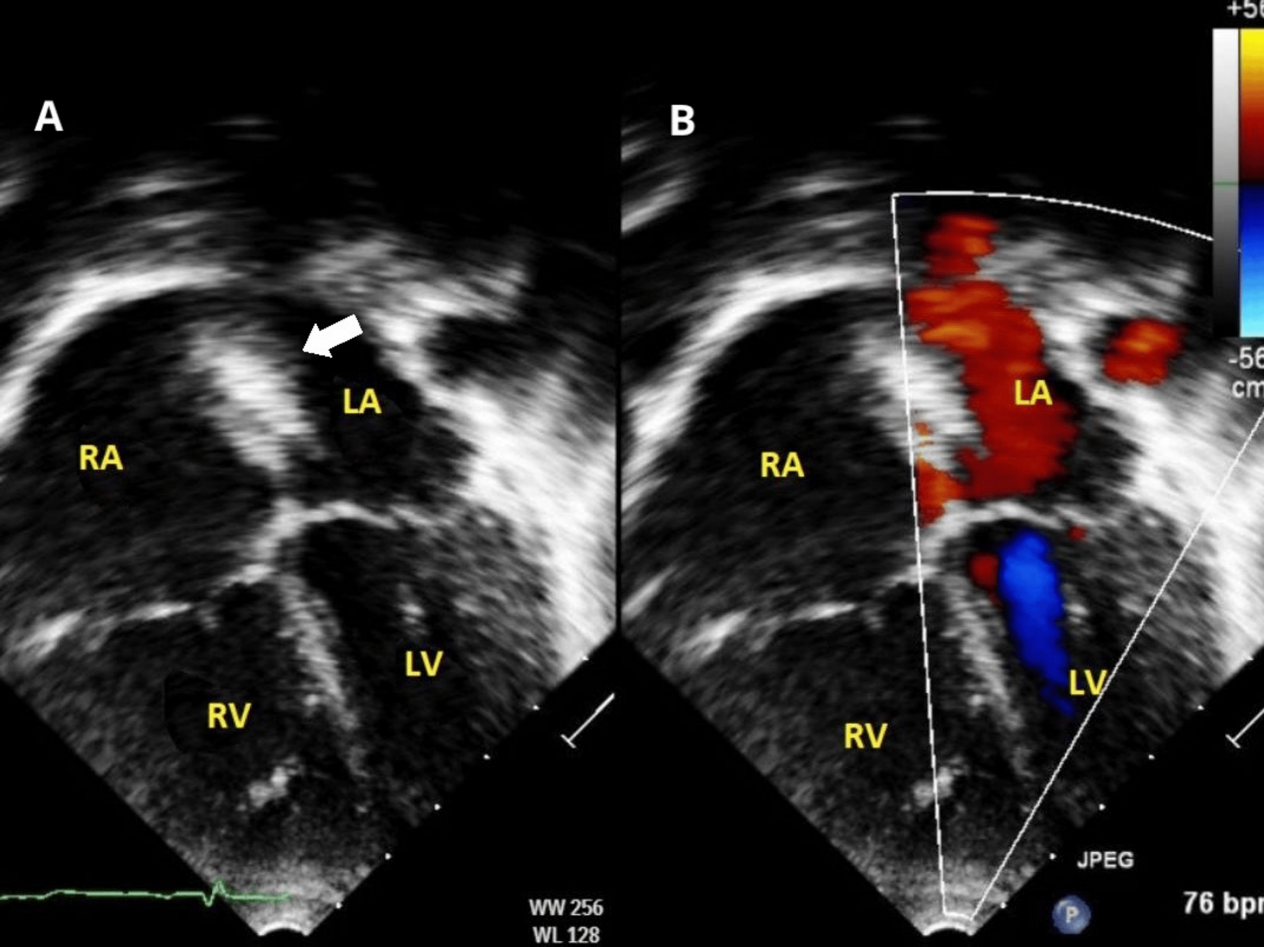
TTE 24 hours after ASD device closure (A) Apical four-chamber view demonstrating the ASD device (arrow) in place with no encroachment on the atrial wall or atrioventricular valves. (B) Colored apical four-chamber view showing no significant residual shunt LA: left atrium; LV: left ventricle; RA: right atrium; RV: right ventricle; TTE: transthoracic echocardiography; ASD: atrial septal defect
